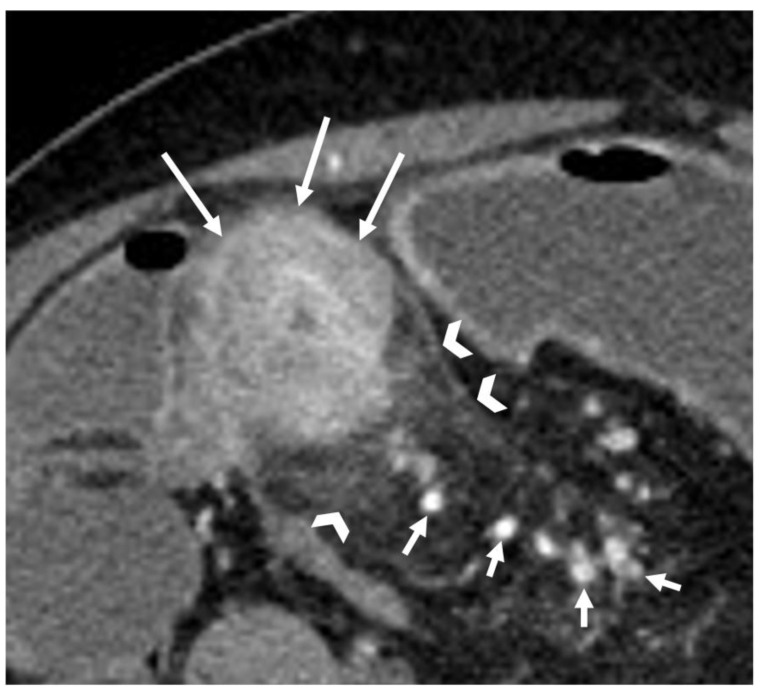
CT appearance of inflammatory strictures. Axial CT image after contrast medium injection shows marked wall thickening of a bowel loop (long arrows), with enlarged vessels (short arrows) in the adjacent mesentery, diffusely hyperdense (arrowheads). These CT features are related to active inflammation.
